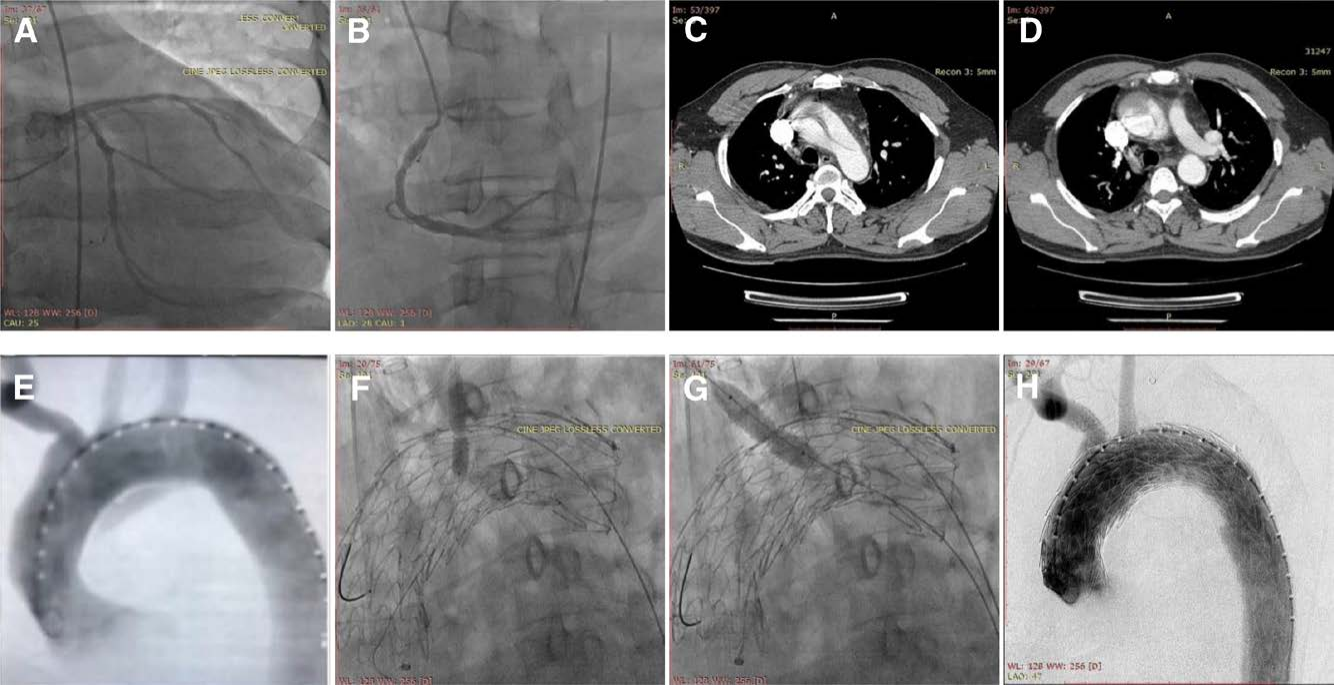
The perioperative images of the thoracic endovascular aortic repair. (A) Coronary angiography showing that 30%–40% of stenosis was in the proximal-middle segment of the left anterior descending artery. (B) Coronary angiography showing that the right coronary artery was not obviously stenotic. (C) CTA showing that the intimal tear started from the distal ascending aorta at the inner curve of the ascending aorta. (D) The distal tear extended to the left subclavian artery. (E) The angiography showing the location of the dissection. (F) The balloon placed for predilatation via the left common carotid artery in a retrograde way. (G) The balloon placed for predilatation via the right common carotid artery in a retrograde way. (H) The aortogram showing the left and right common carotid arteries were patent without any endoleak, but the left subclavian artery failed to reconstruct. CTA = computed tomography angiography.
